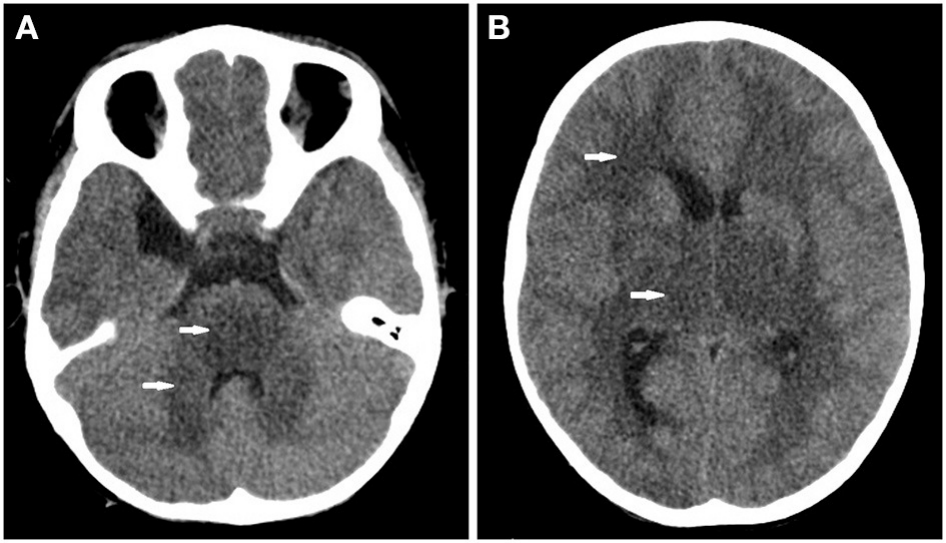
Cranial CT found (A) extensive lesion of low intensity in the pons and cerebellum (white arrow) and (B) the bilateral white matter and thalamus (white arrow).
